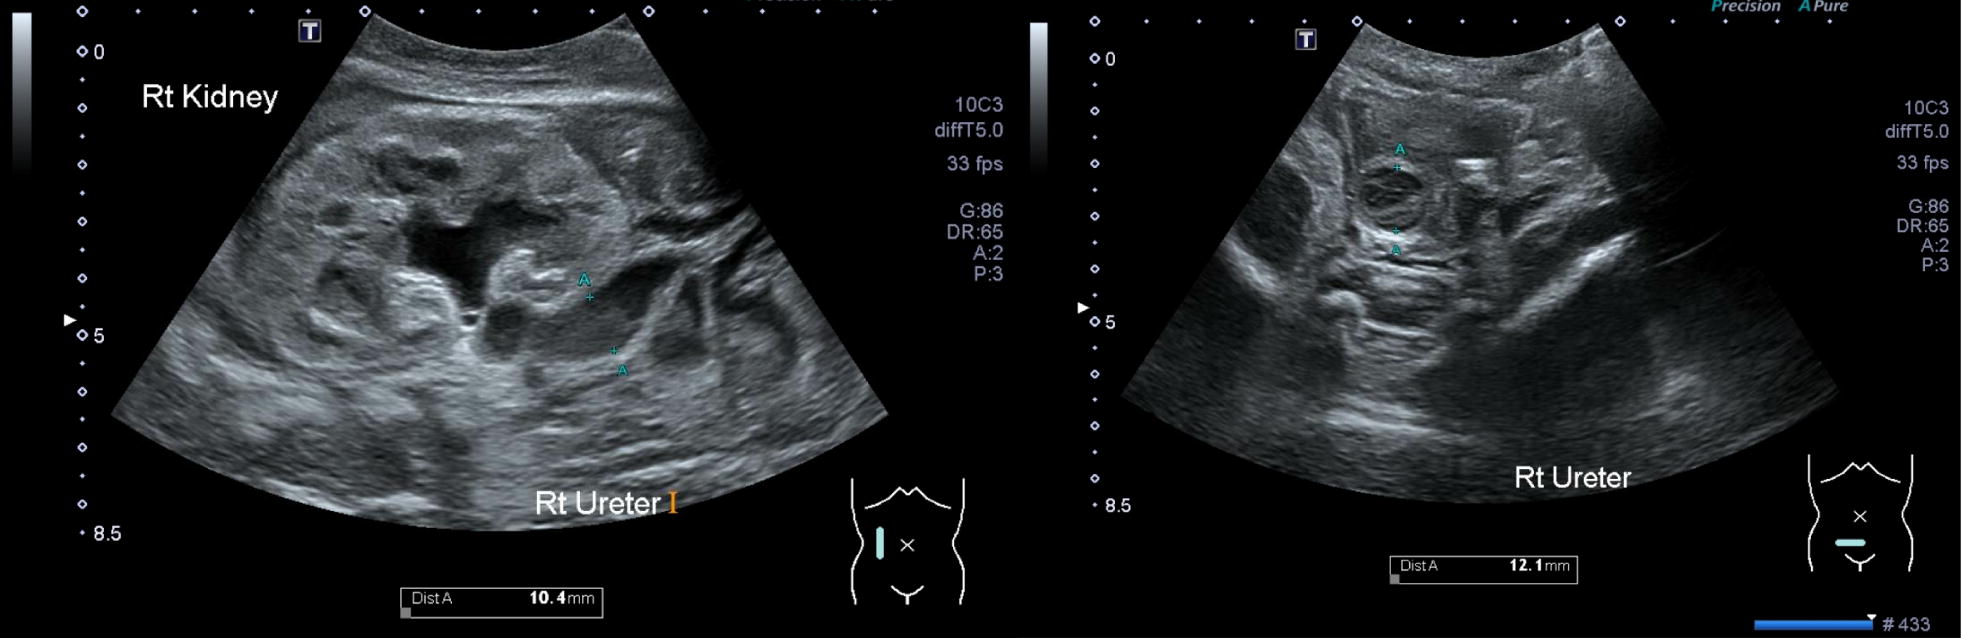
Renal tract ultrasound showing appearance of hydro-nephrosis of right kidney and dilated right ureter (diameter 12.1 mm) on initial presentation. *Rt Kidney* right kidney, *Rt Ureter* right ureter, *Dist A* distance A (12.1 mm)
